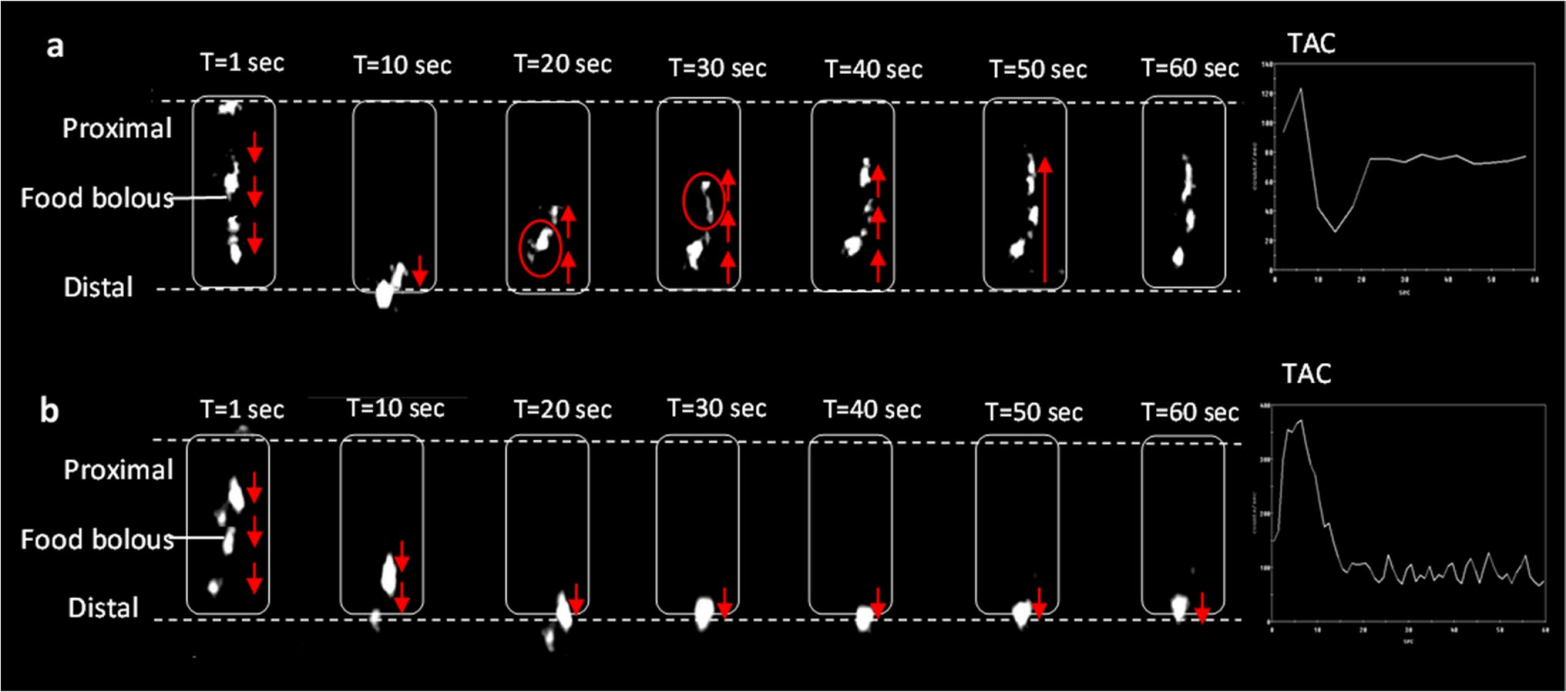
Bolus reflux on 60-s nuclear scintigraphy esophageal swallow study. Upper dotted line = manubrium, lower dotted line = xiphisternum. a Bolus-induced deglutitive reflux pattern. b No reflux
